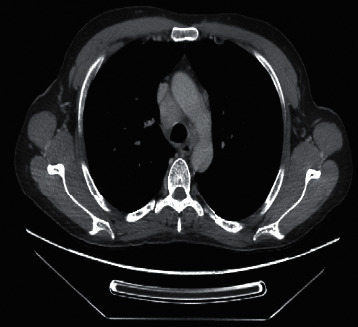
A CT scan of the chest (mediastinal window) showing enlarged right paratracheal lymph node measuring 18 mm in short axis.
